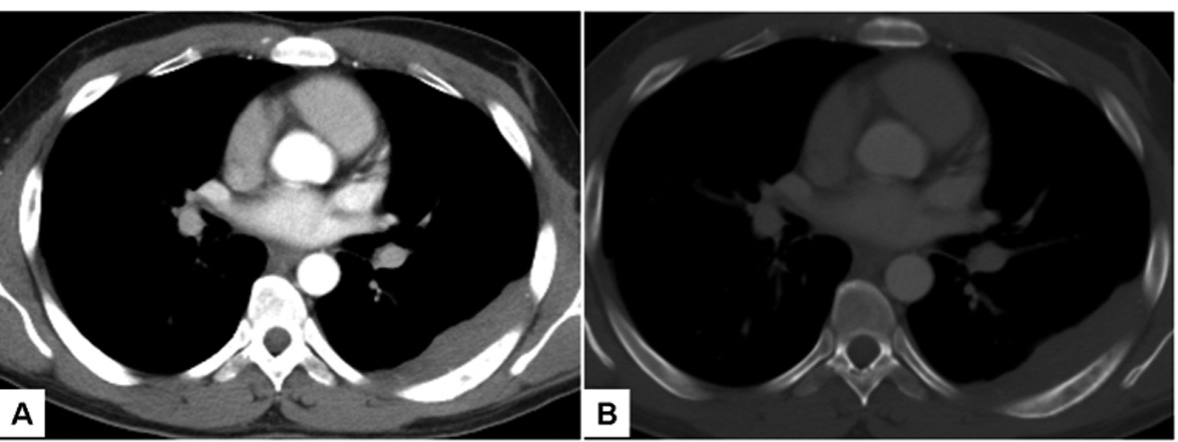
Axial CT images through mid-thorax demonstrate pleural-based soft tissue mass along the posterior aspect of the left hemithorax (A). On bone window (B), permeative destruction of the adjacent 6th rib is noted.
